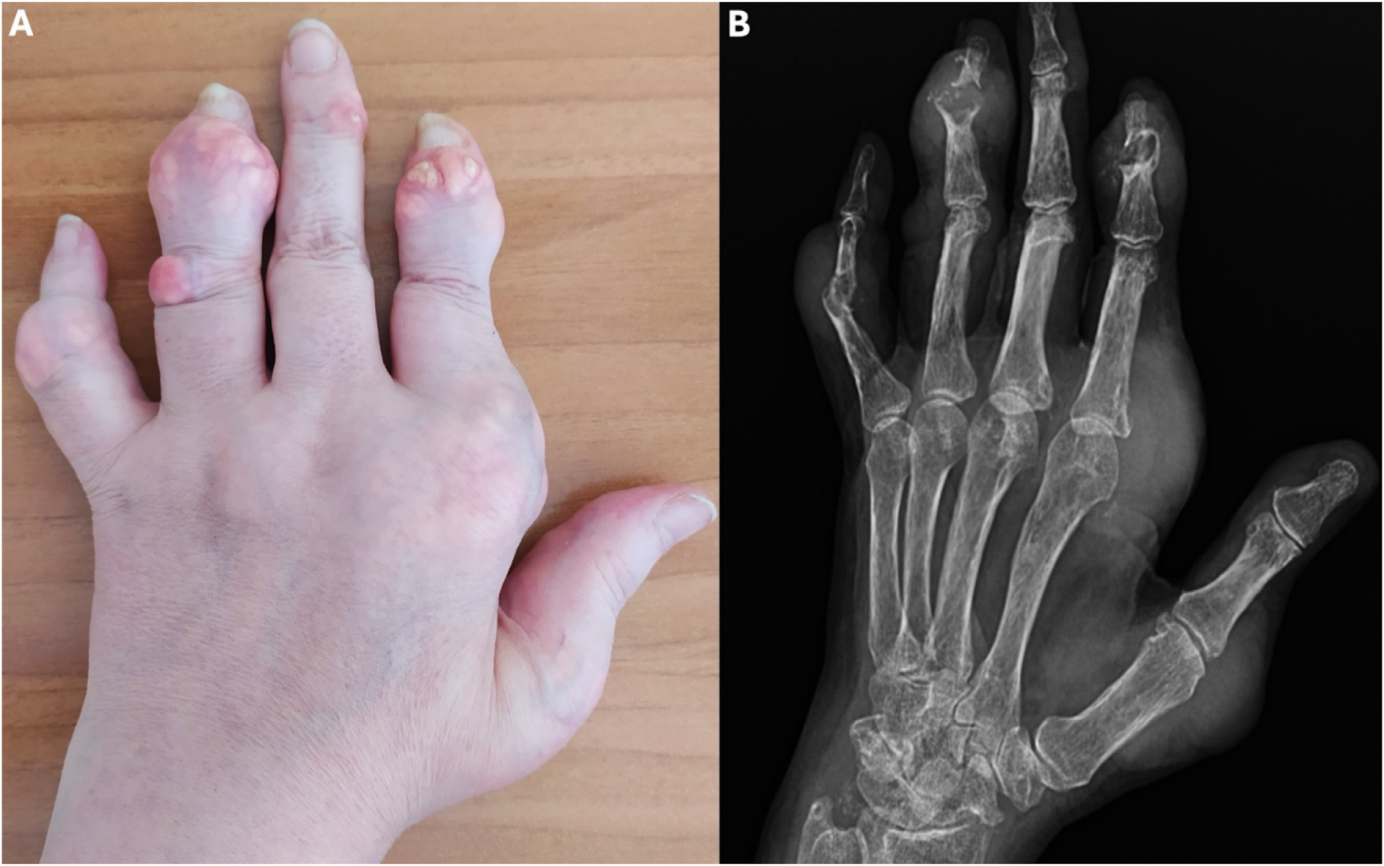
Tophaceous gout. Comparison of findings detectable on physical examination (Panel A) and conventional radiography (Panel B). Please note the yellow/white appearance of tophi in panel A and the extensive bone destruction around them (eg second and fourth distal interphalangeal joints) in panel B. Written permission was obtained from the patient for using the images in a publication.
